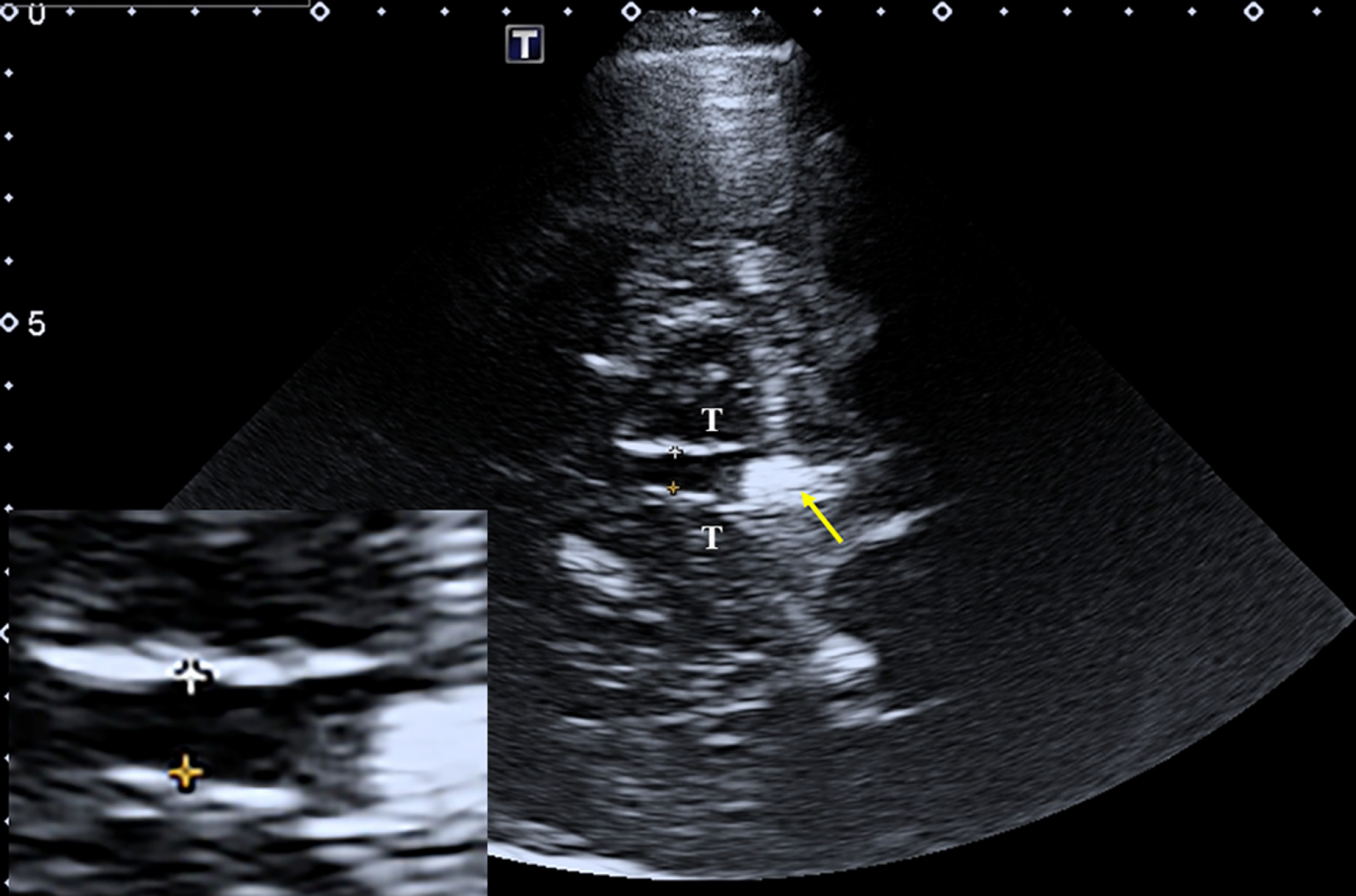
TCS images of the scanning planes at the level of the thalamus in a PD patient. TCS image of axial section at the level of the thalamus. The yellow arrow indicates the pineal gland that can be regularly observed on TCS as a “sonographic landmark” of high echogenicity owing to calcification. T, thalamus; TCS, transcranial sonography.
